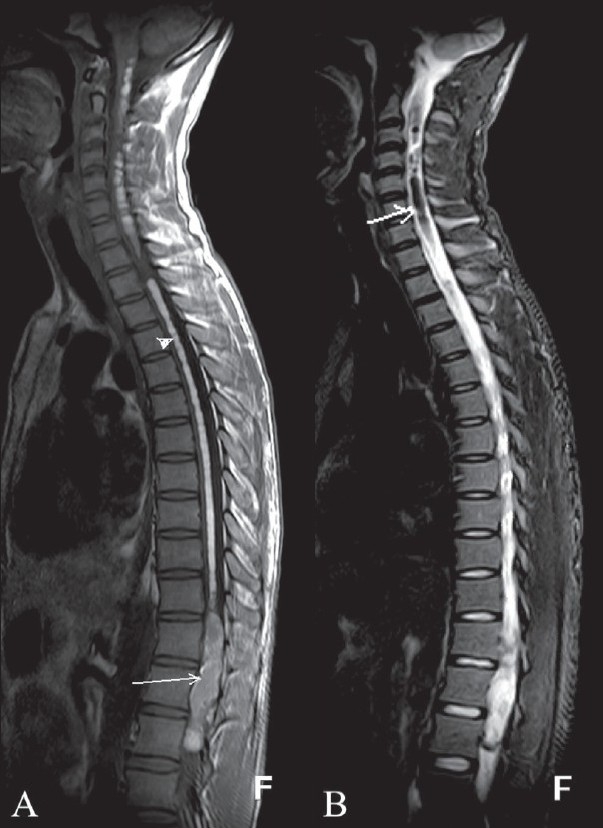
T1W (A) sagittal MRI image shows a heterogeneous intramedullary lesion (arrow) causing expansion of the conus medullaris region with hyperintense intramedullary signal (arrowhead) extending from the cervical to the lumbar regions. The sagittal T2W fat-saturation image (B) shows suppression of signal (arrow) in the upper spinal cord.
